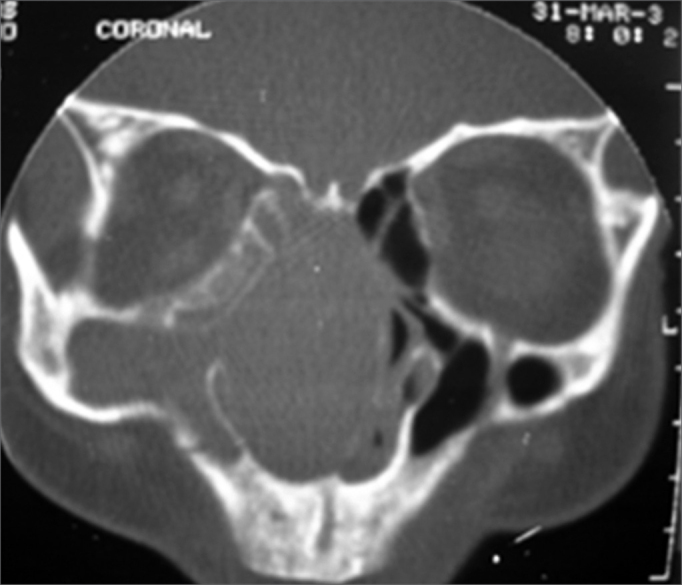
Computed tomography of the paranasal sinuses - coronal plane. Soft tissue attenuation material occupies the nasal fossae, the right maxillary sinus, and some ethmoid cells. The cartilaginous septum is deviated to the left. There is no bone destruction.
